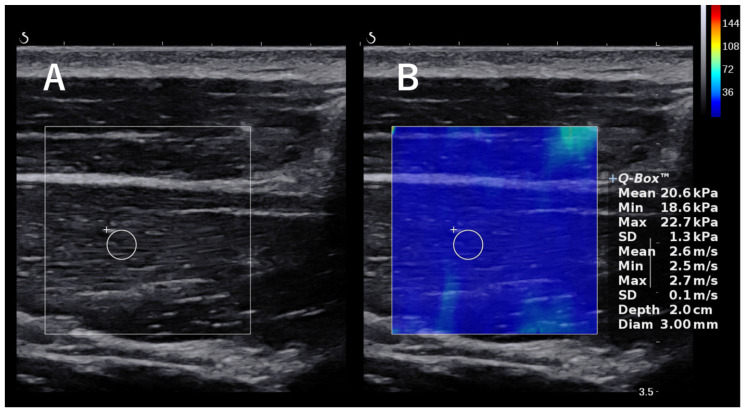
The images of shear wave elastography. The circle in the figure is the region of interest. (A) B-mode ultrasound image showing the supraspinatus muscle. (B) B-mode image with overlaid elastogram. The circular region of interest was set near the central part of the muscle.
